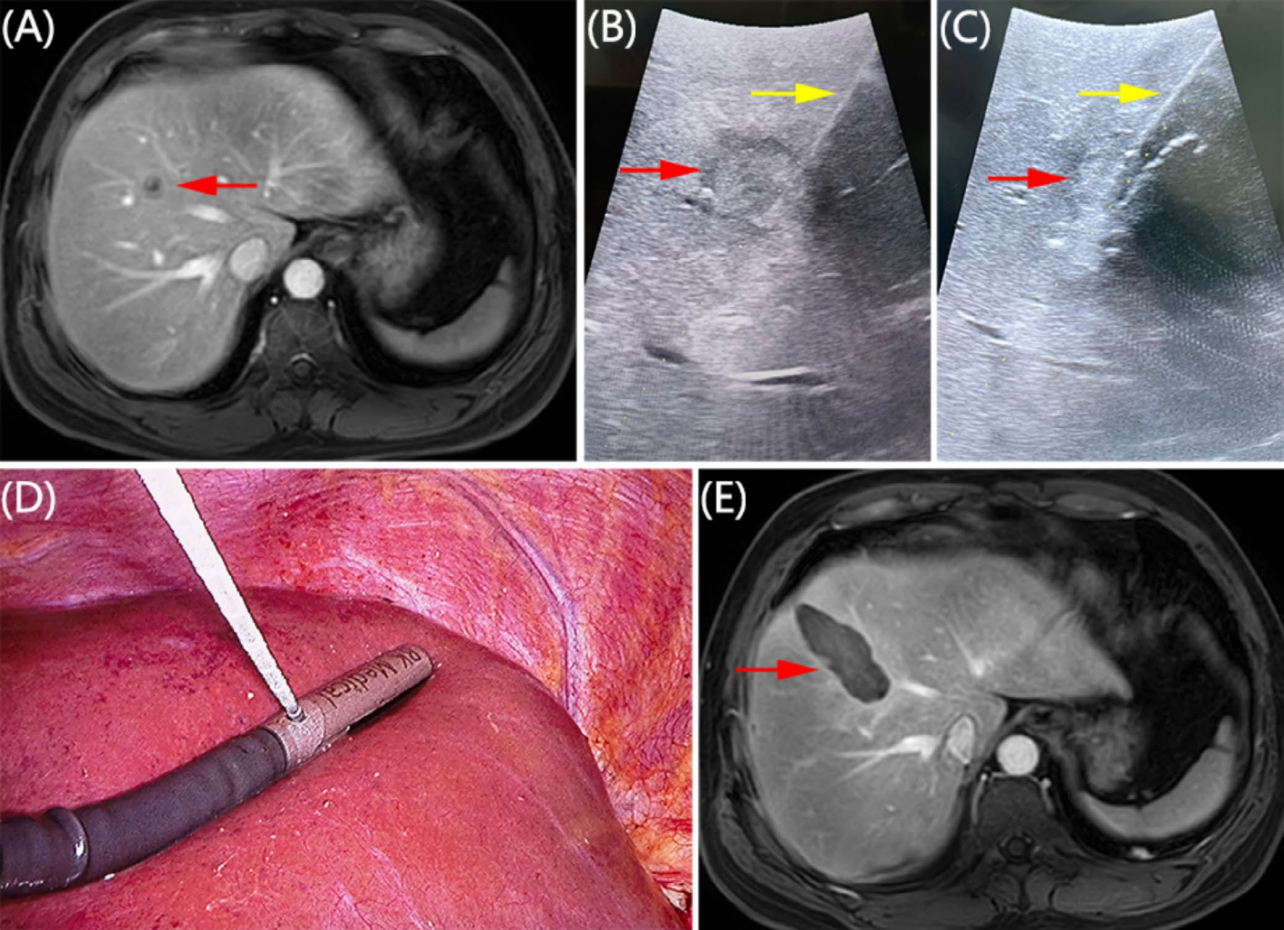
Example of using ultrasound‐guided MWA for treating HCC are located in the Junction of Segments 5 and 8 of liver (adjacent to middle hepatic vein). (A) A single nodule (indicated by the red arrow) was visible on the preoperative MRI scan. (B and C) The needle (yellow arrow) was placed at the left and right side of the tumor center because the nodule(red arrow) was 2.1 cm in diameter and adjacent to the middle hepatic vein. (D) Insertion of the antenna into the nodule was conducted under laparoscopic ultrasound guidance. (E) The tumor was entirely ablated, as evidenced by the postoperative MRI scan.
